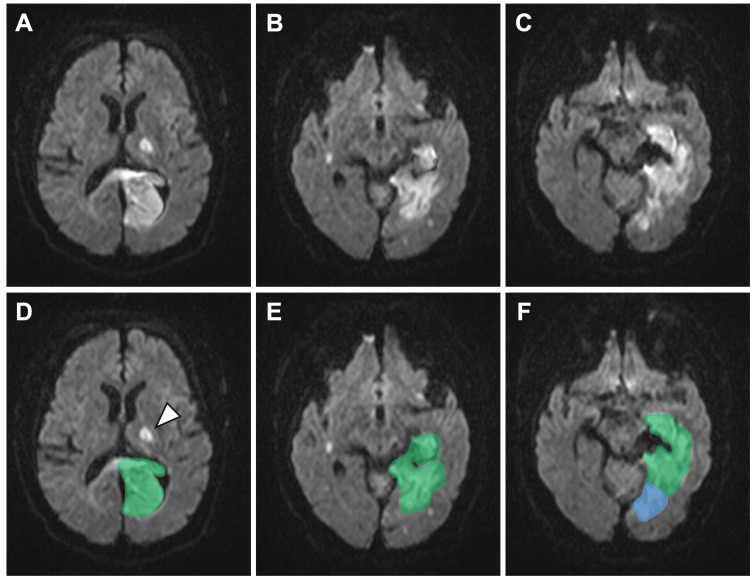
MRI on postoperative day 6 (A-C) Axial diffusion-weighted MRI obtained on postoperative day 6 demonstrates acute infarctions in the lateral thalamus within the thalamogeniculate artery territory and in the medial temporal and occipital lobes. (D-F) Territorial labeling of the infarctions corresponding to (A-C). The white arrowhead indicates infarction in the left lateral thalamus. The green-shaded region represents the medial temporal lobe, corresponding to a PCA-MCA border zone, and the blue-shaded region represents the occipital lobe, corresponding to the primary PCA territory. PCA: posterior cerebral artery; MCA: middle cerebral artery; MRI: magnetic resonance imaging
